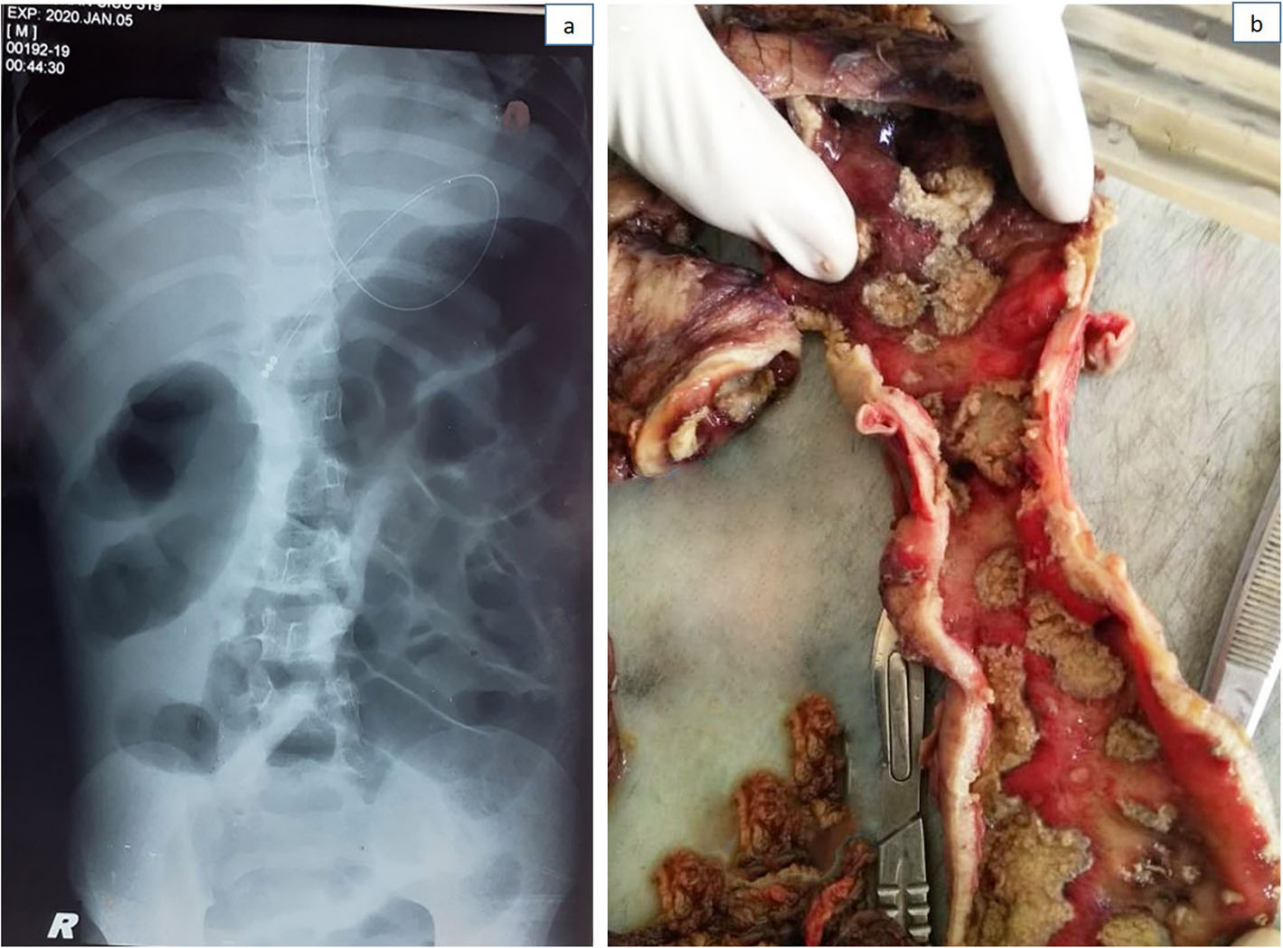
a X-ray image of the patient’s abdomen. The dilated bowel loops can be seen. b Section of the colon showing widespread amebic ulcers
